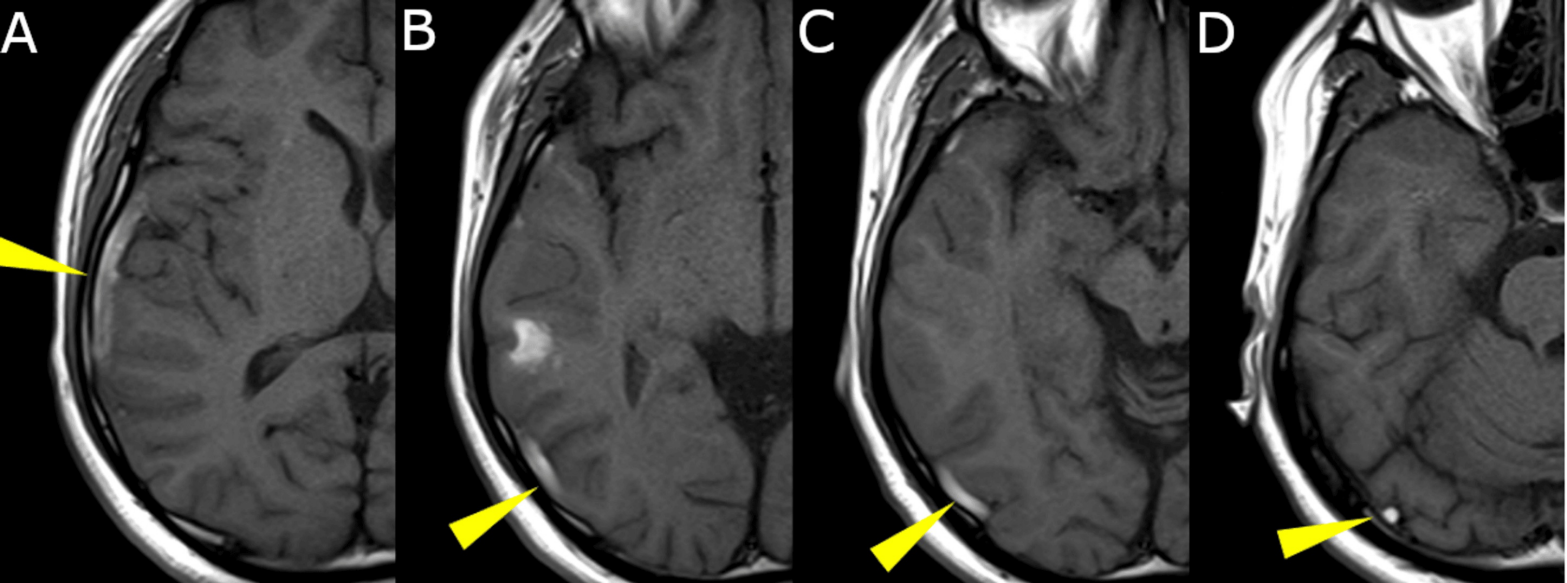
T1-weighted MRI revealing a linear hyperintense signal along the right vein of Labbé Axial T1-weighted MRI slices (A-D) demonstrate a continuous linear hyperintense signal along the anatomical course of the right vein of Labbé (yellow arrowheads), consistent with a subacute venous thrombus. The signal is most pronounced in slices A and B, corresponding to the right temporal convexity, and gradually attenuates posteriorly in slices C and D. In addition, a focal area of subcortical hyperintensity in slice B suggests a small subcortical hemorrhage, which may represent hemorrhagic transformation secondary to venous congestion.
